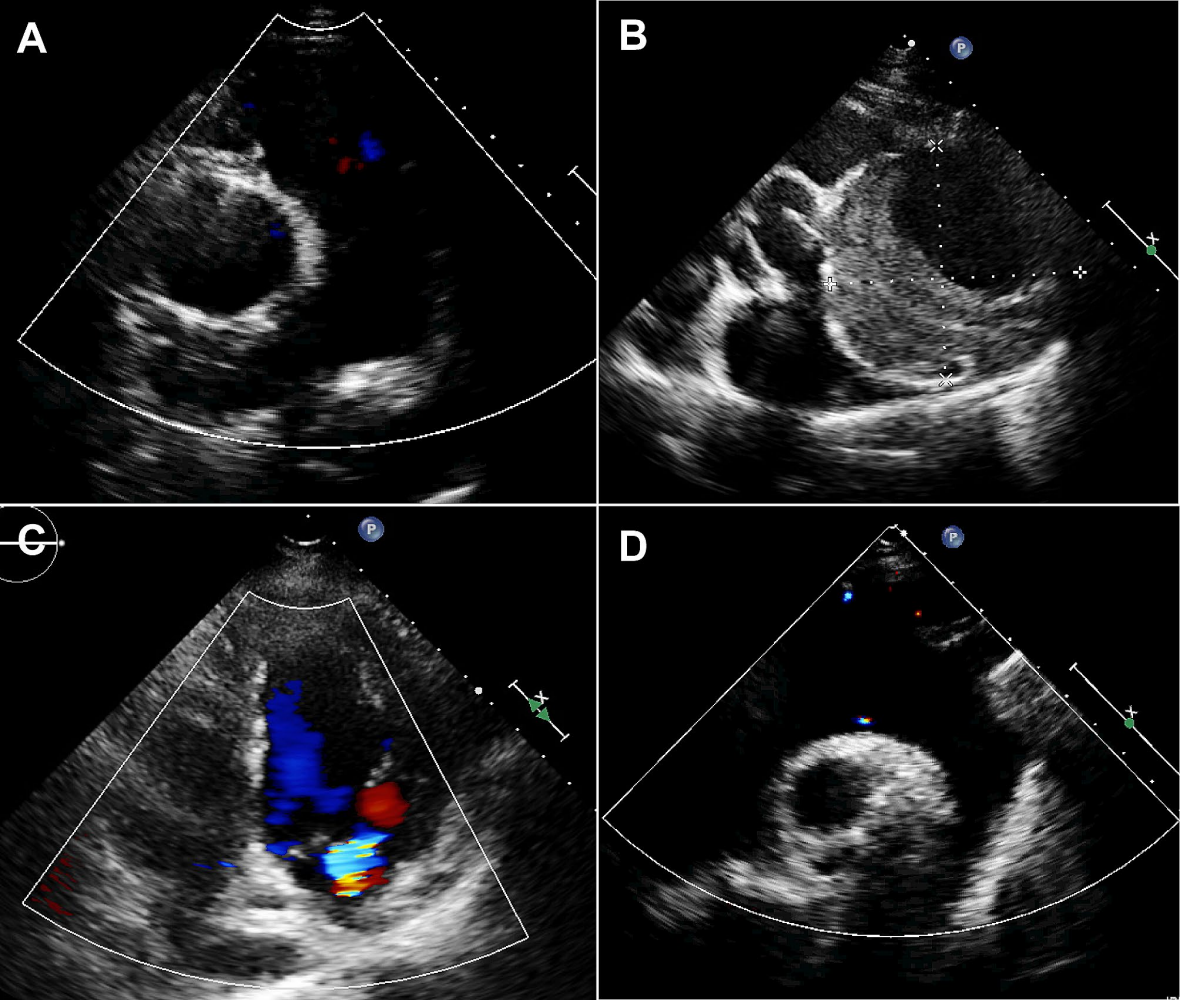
(A, B) A solid-cystic mass can be seen outside the left coronary sinus of the aorta in echocardiography. (C) No blood flow in the isoechoic area of the mass. (D) The contrast agent enters the cystic cavity after continuous interruption, and the enhancement in the isoechoic area is not obvious; it is ultimately diagnosed as a huge coronary artery aneurysm and concurrent thrombosis
